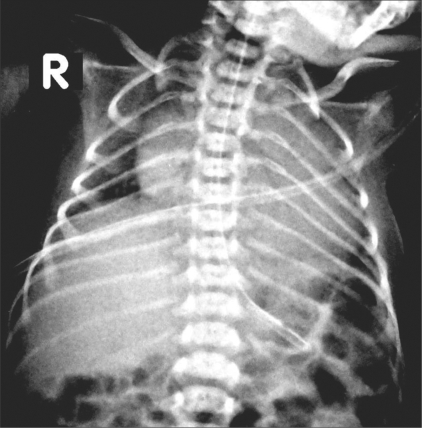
Chest radiograph on day 1
